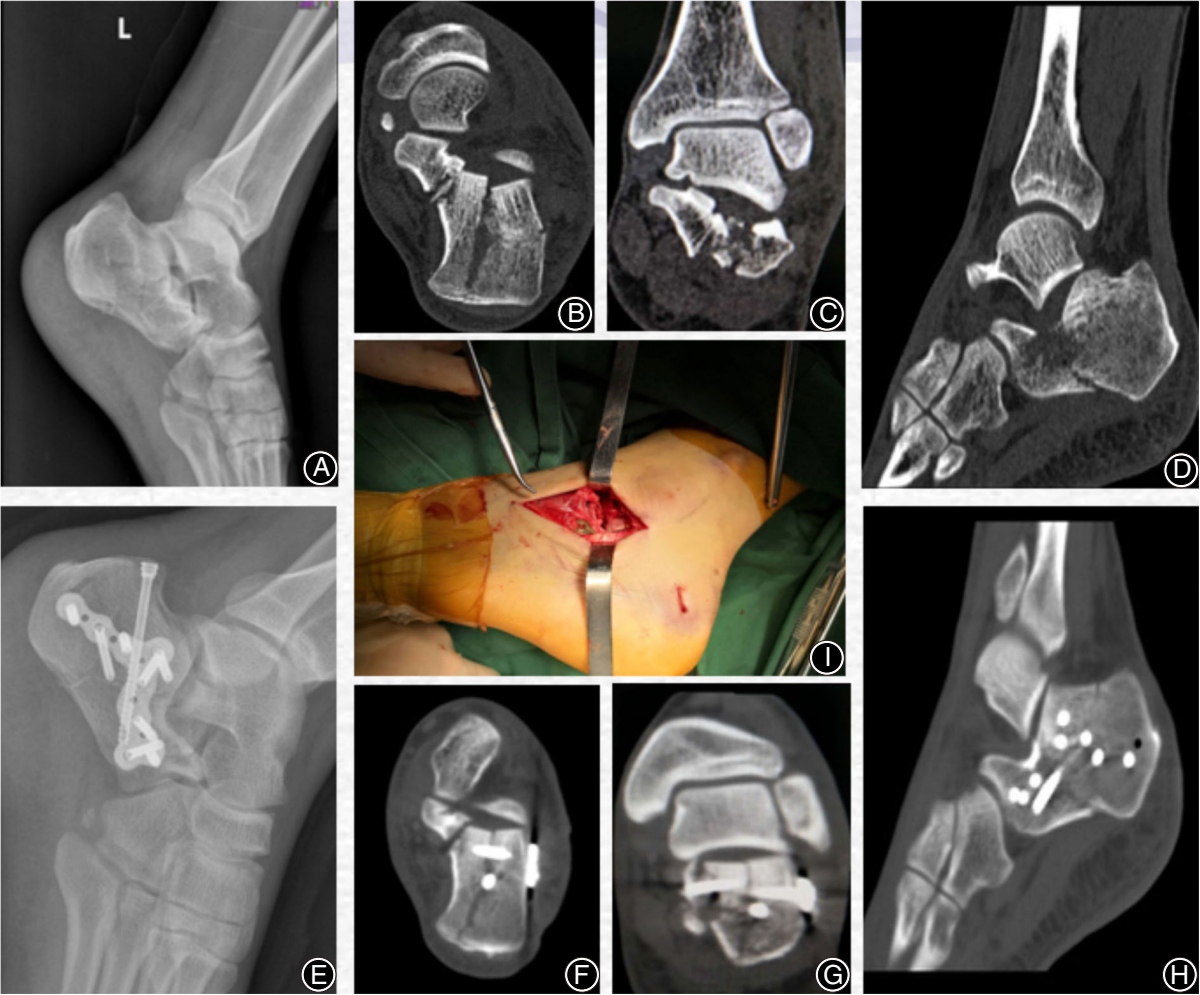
A 44‐year‐old female patient fell from height, resulting in an intra‐articular calcaneal fracture. (A) Preoperative lateral X‐ray. Preoperative CT scan shows (B–D): A Sander type III AB calcaneal fracture with a subtalar articular surface collapse and loss of Bohler's angle and Gissane's angle. (E) Postoperative lateral X‐ray. (F–H) Postoperative CT scan shows recovery of the subtalar articular surface, Bohler's angle, and Gissane's angle. (I) Intraoperative incision picture.
